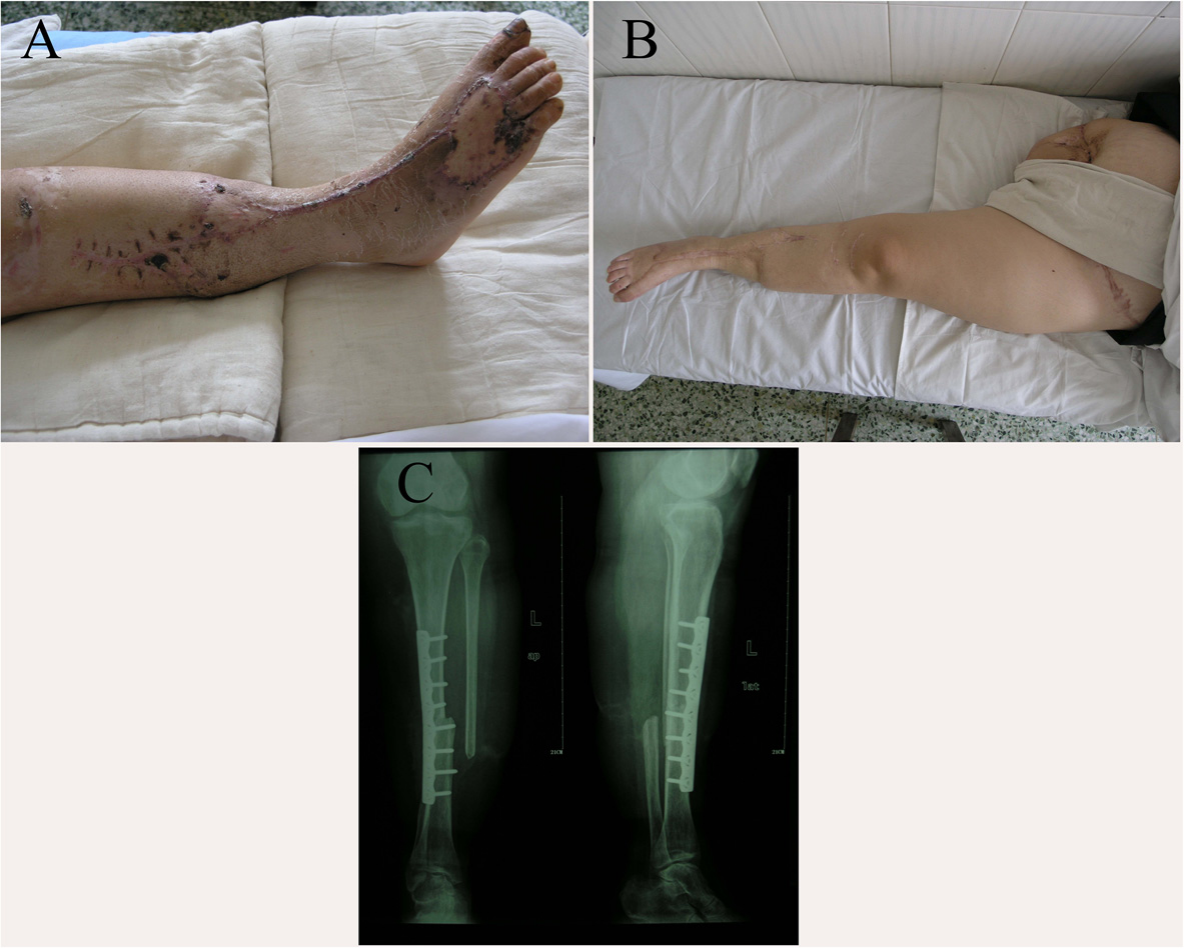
Post-operative status of our patient. (A) One month post-operatively. (B) Three years and three months post-operatively. (C) X-ray image taken at 12 months post-operatively.
